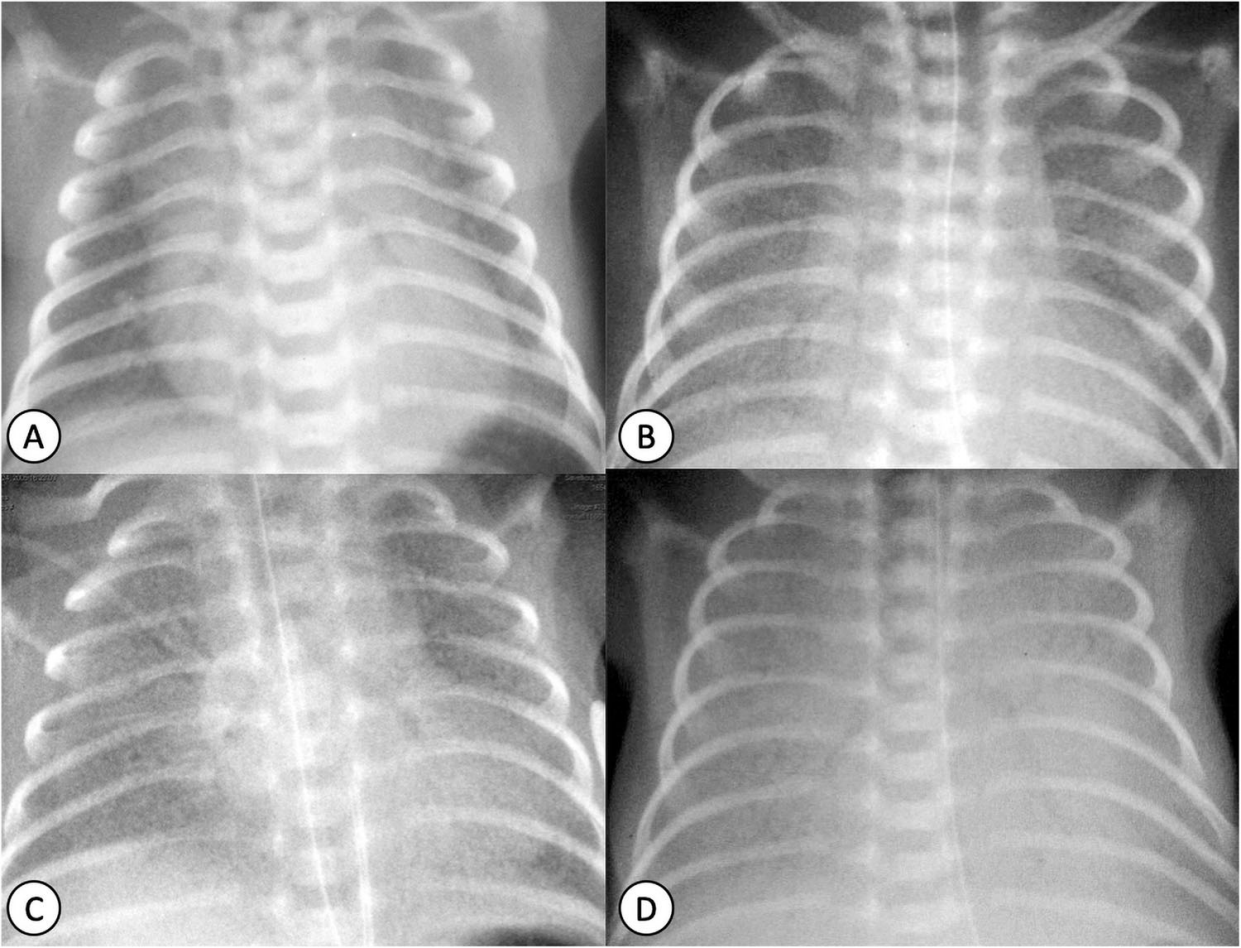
Respiratory distress syndrome grading on chest radiographs. A RDS grade 1: a ground-glass appearance of the lungs with well-expanded lung and with limited air bronchogram, (B) RDS grade 2: more prominent opacification of the lung, which is relatively well-expanded, but with increased presence of air bronchogram and still well distinct heart and diaphragmatic contours. C RDS grade 3: coarse granular opacification of the lungs and less distinct cardiac and diaphragmatic silhouette, widespread air bronchograms and poorly expanded lungs and (D) RDS grade 4: total collapse of the lungs with complete opacification, indistinct cardiac and diaphragmatic silhouettes and widespread distinct air bronchograms
